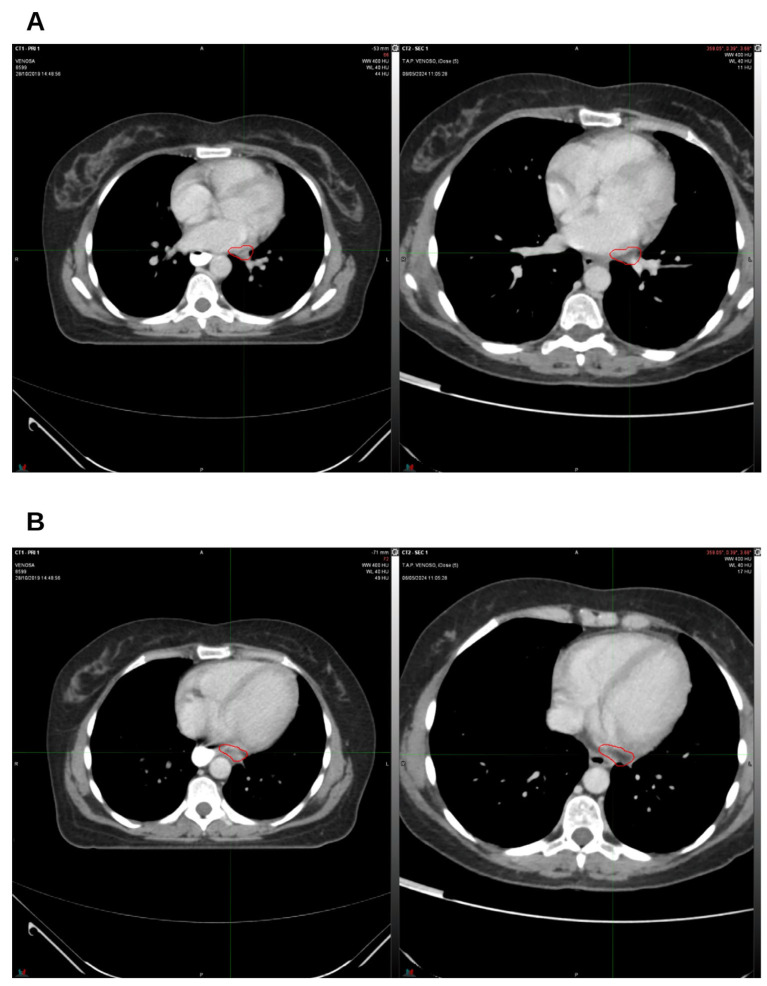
The last follow-up. On the left, CT from October 2019, pre-radiation therapy treatment; on the right, CT from May 2024. (A) Hilar lymph node; (B) left para-aortic lymph node. The indicated region highlights the boundary of the treated target.
